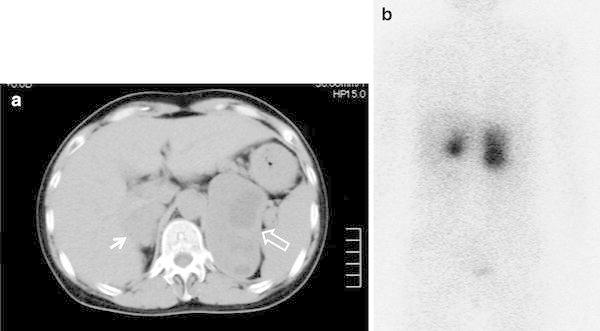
a Computed tomography of abdomen shows *right* (*arrow*) and *left* (*open arrow*) adrenal tumors. b
